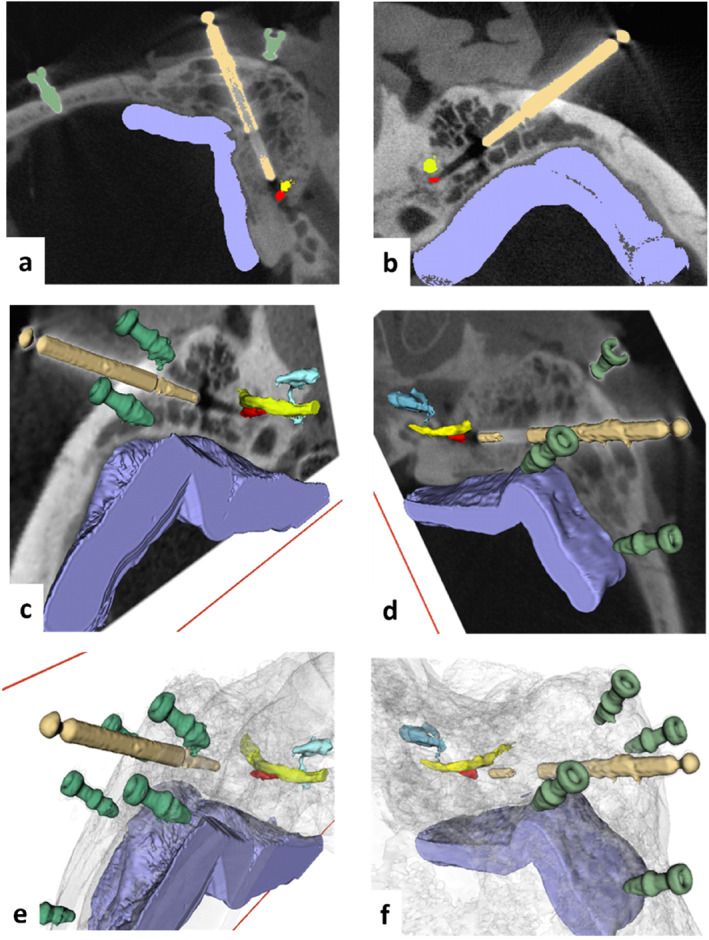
Results of the drilling session. Panels (c), and (e) show the results from the left side. Panel (b), (d) and (f) are the results from the right side. Panels (a) and (b) show the linear trajectory reaching the stapedius muscle. Panels (c) and (d) show the same CT slices of (a) and (b) overlayed on the 3D post‐drilling reconstructions. In panels (e)and (f), the bone is overlayed on the 3D reconstruction to give a complete overview. The segmented structures are coloured as follows: beige = surgical rod inserted for the post‐surgical CT scan; red = stapedius muscle; yellow = facial nerve; lilac = sigmoid sinus; green = fiducial screws; and light blue = ossicles.
